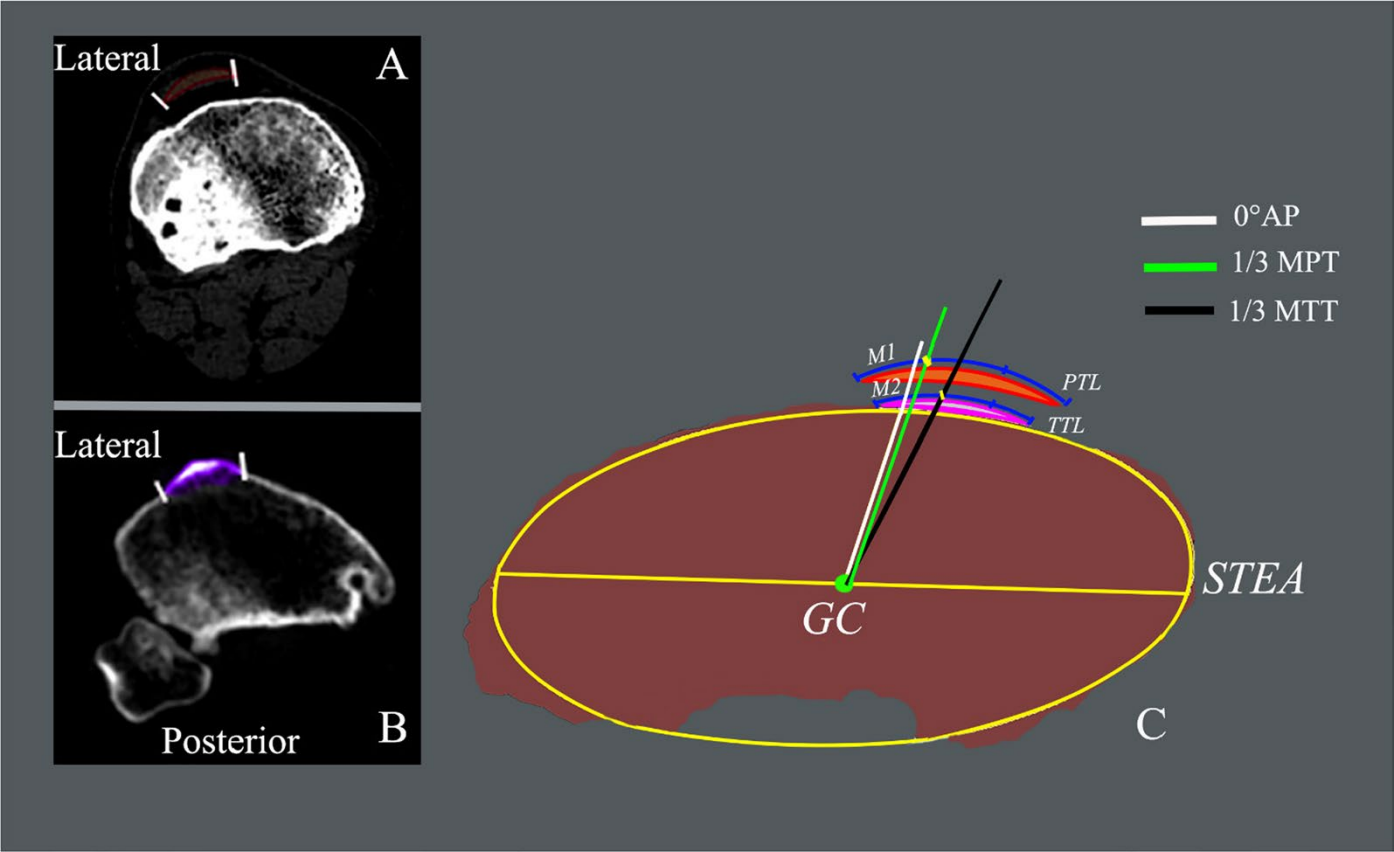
A, B Patellar tendon and tibial tubercle on CT axial cut. C The angle between the 1/3MPT, 1/3MTT axis, and the 0°AP axis was defined as the rotational mismatch angle. PTL; patellar tendon length. TTL; tibial tubercle length. M1: width of patellar tendon on the medial side of 0°AP axis. M1/PTL; the medial patellar tendon width percentage. M2; width of the tibial tubercle on the medial side of 0°AP axis. M2/TTL; the medial tibial tubercle width percentage. GC; geometric center. 1/3MPT; medial one-third of the patellar tendon. 1/3MTT; medial one-third of the tibial tubercle
